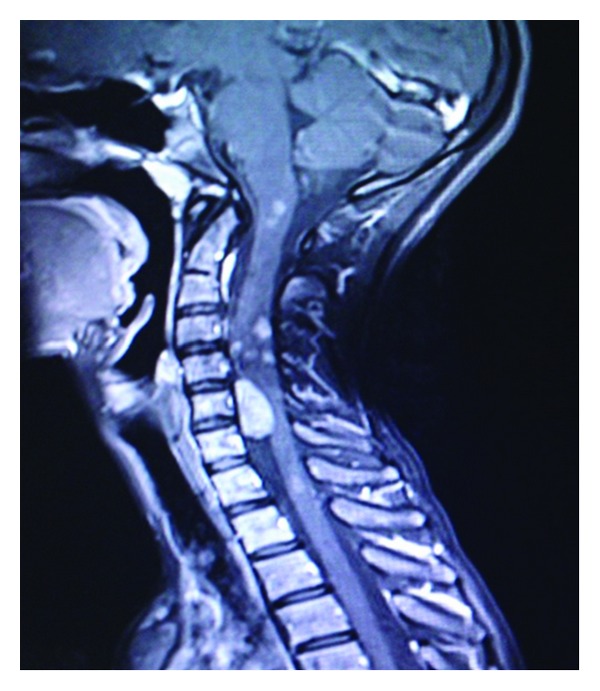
Sagittal T1 postcontrast MR image of the cervical spine showing multiple intramedullary intensely enhancing mass lesions at cervical cord and extramedullary mass lesion at C5 level suggestive of ependymomas/astrocytomas and neurofibroma, respectively.
